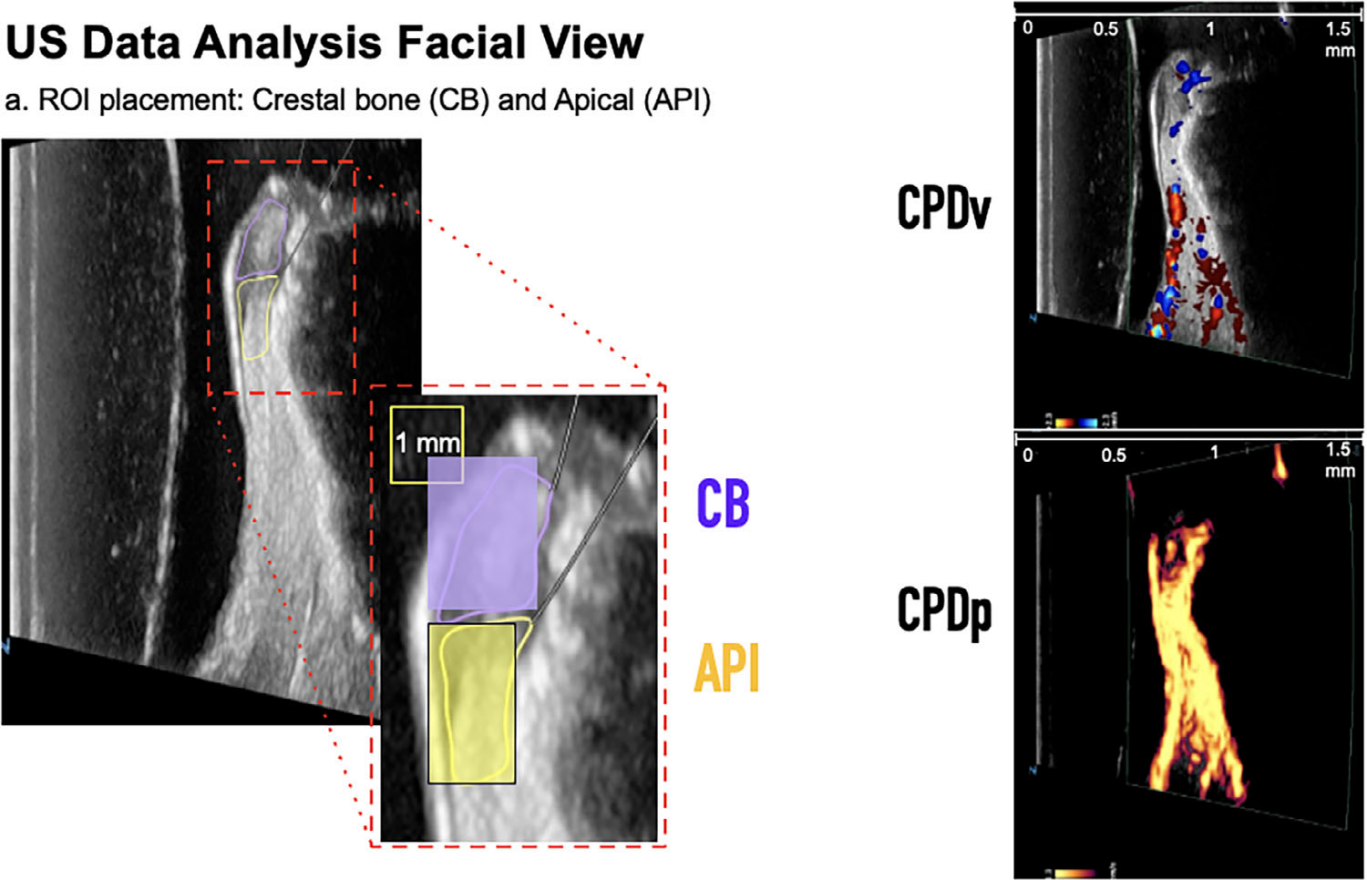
Same case was imaged from the facial view with ultrasound (US). B‐mode images (left), color flow velocity (right top) and power images (right bottom) were obtained from facial views, with color flow velocity recorded at 90 dB B‐mode gain cine‐loop and color flow power mode at 40 dB B‐mode gain cine‐loop. Two regions of interest (ROIs) were defined as an example: crestal bone (CB) and apical (API), and color pixel density was calculated to indicate blood perfusion.
